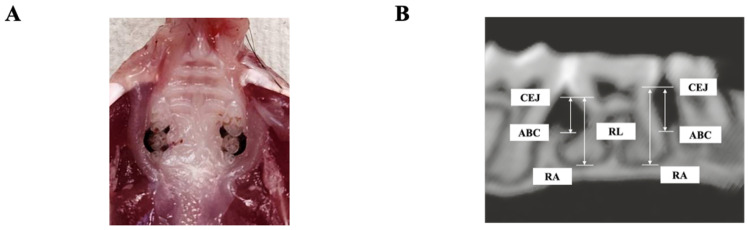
Ligation-induced experimental periodontitis and measurement points in micro-computed tomography images. Alveolar bone loss relative to root length around maxillary second molars in experimental periodontitis is shown. (A) Macroscopic photograph showing silk ligature around the cervical region of the maxillary second molar. (B) Method for analyzing alveolar bone resorption in micro-computed tomography images. The ratio of residual alveolar ridge bone to root length was calculated using the formula (ABC − RA)/RL, where (ABC − RA) denotes the distance from the ABC to the RA, and RL denotes the distance from the CEJ to the RA. CEJ, cementoenamel junction; ABC, alveolar bone crest; RA, root apex; RL, root length.
